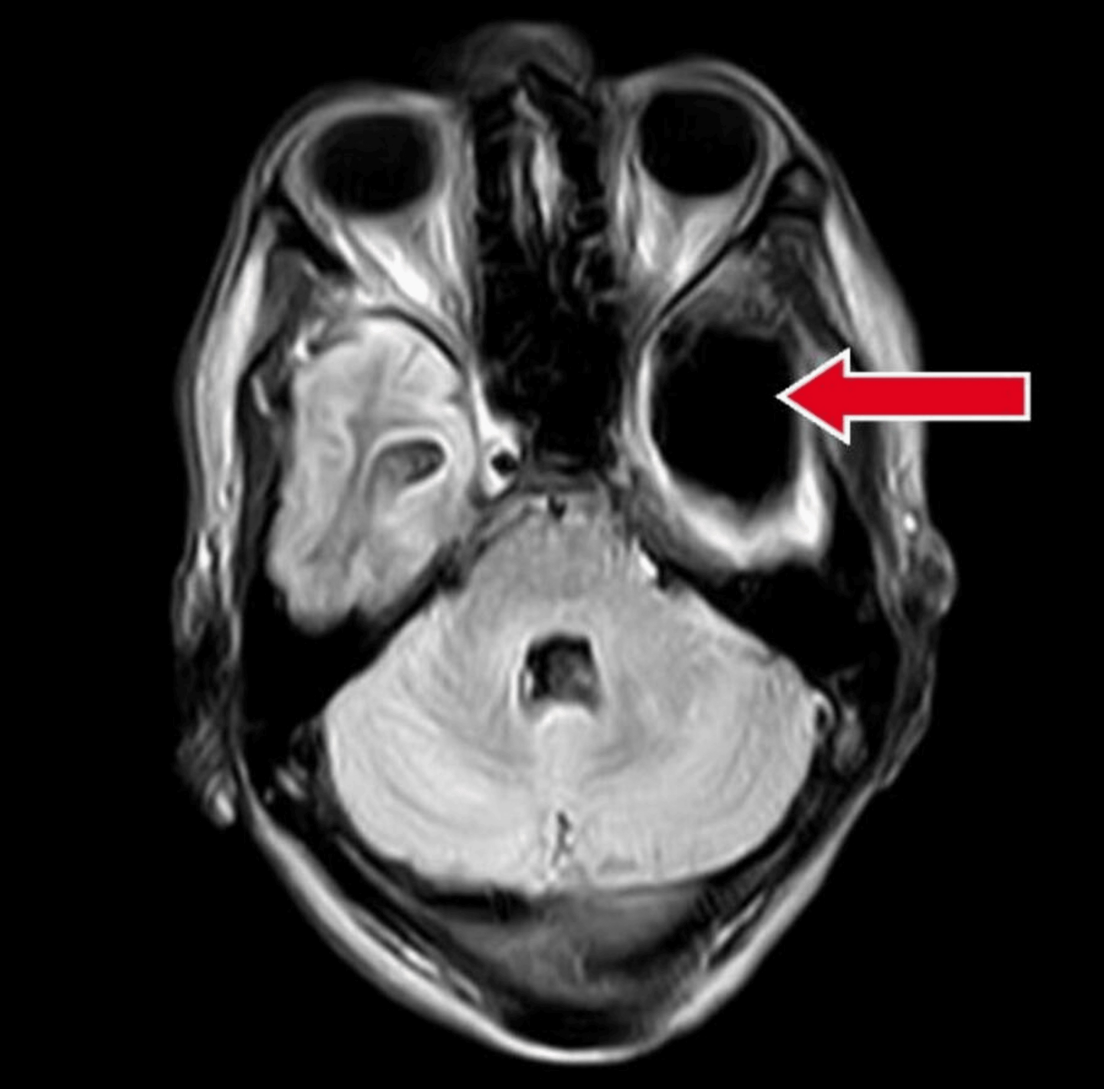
Contrast enhanced magnetic resonance imaging showing porencephalic cyst with red arrow.
